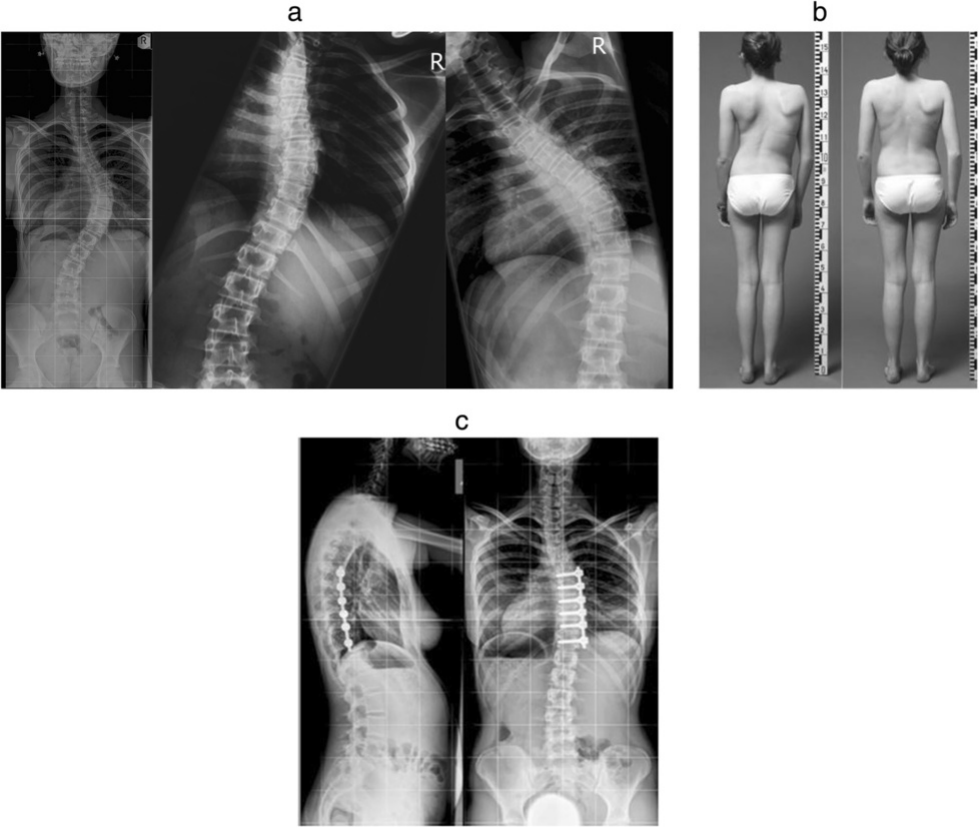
a: A single thoracic curve (Lenke 1). Disc T11/T12 opens in supine bending. T11 is distal LIV. In anteroposterior standing radiograph the apex is disc T8/T9. There are three vertebrae (T9, T10,T11) distal to the apex and therefore three proximal vertebrae (T6, T7, and T8) need to be included in the proximal arm. b: Radiographs and clinical pictures preoperatively and at 5-year follow-up.
